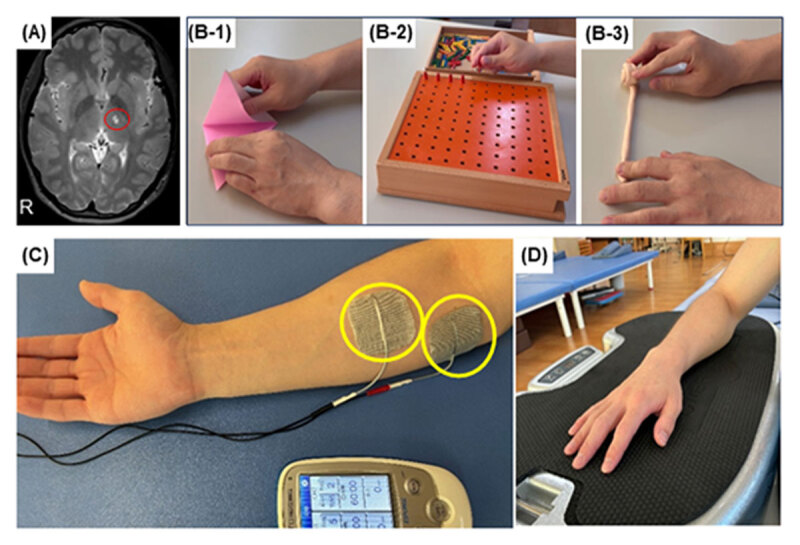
Thalamotomy target and multimodal rehabilitation components. (A) Postoperative axial T2-weighted magnetic resonance imaging showing a coagulation lesion (red circle) within the left ventro-oralis/ventral intermediate nucleus border zone following stereotactic thalamotomy. (B) Components of fine motor training: (B-1) “origami,” a Japanese paper-craft art (10 min; such as square box and crane); (B-2) pegboard tasks (13 min; such as single-fingertip rotation and grasp-and-hold); (B-3) putty exercises (7 min; including stretching and pressing, rolling). Task difficulty was progressively adjusted to the patient’s performance. (C) Transcutaneous electrical nerve stimulation: Surface electrodes were placed over the muscle bellies of the right flexor carpi radialis and flexor carpi ulnaris. Stimulation parameters: 50 Hz, 250 μs pulse width, and 6 mA (sensory-level intensity); device: ITO ESPURGE (ITO Co., Ltd., Kawaguchi, Japan). (D) Vibratory stimulation to the right upper limb: five 1-minute bouts at 35 Hz, with the right hand and distal forearm placed on a vibrating platform (Personal Power Plate; Performance Health Systems, Northbrook, IL, USA). Abbreviations: Vo, ventro-oralis nucleus; Vim, ventral intermediate nucleus.
